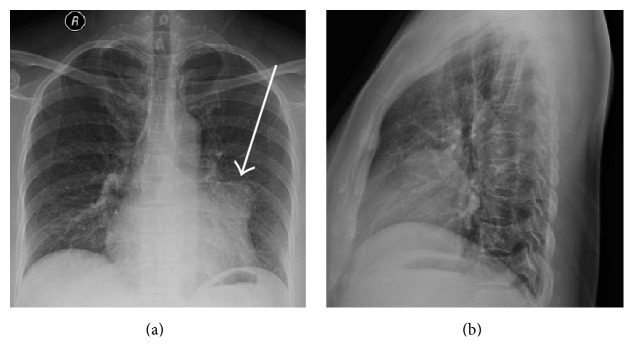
Chest radiography showed small opacity in the left lower lobe (arrow).
